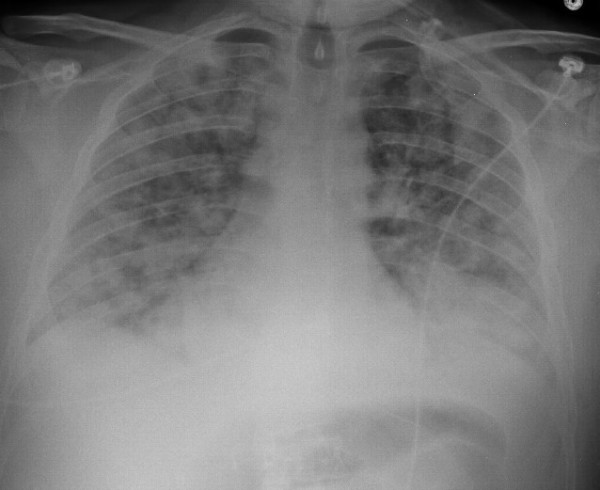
Chest radiograph showed bilateral pulmonary infiltrates.
